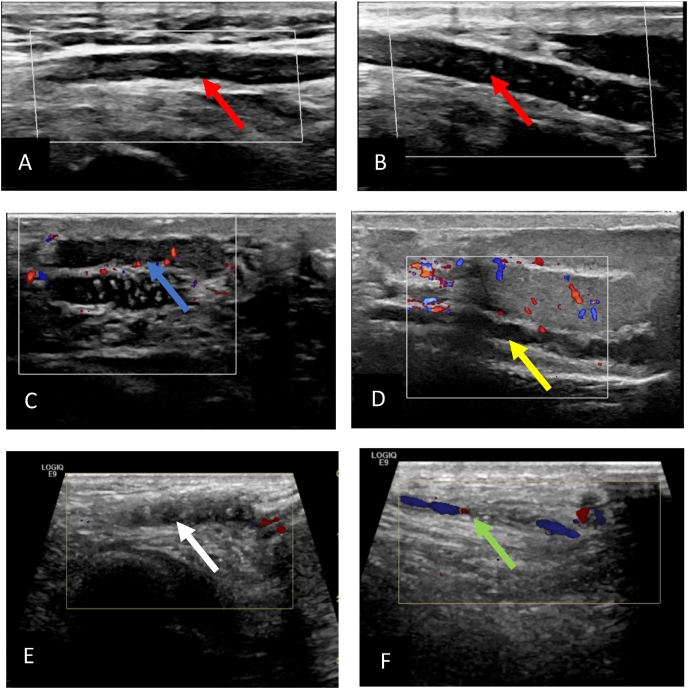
Doppler ultrasound showing A and B showing left spermatic vein thrombosis with flow on (Red arrow), C: left spermatic vein thrombosis on follow up images (blue arrow), D right spermatic vein thrombosis (yellow Arrow), E: perisistance of left pseramtoc vin thrombosis after 6 month of anticoagulation (white Arrow) while F showing resolution of the right spermatic vien thrombosis with flow on doppler images after antigoagulation (green arrow). (For interpretation of the references to colour in this figure legend, the reader is referred to the Web version of this article.)
